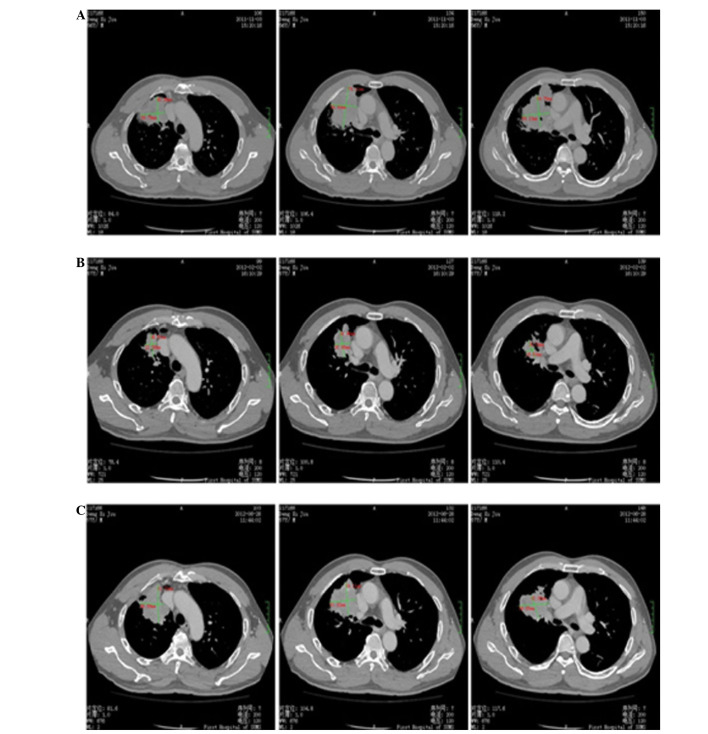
Computed tomography scans of the present non-small cell lung cancer patient. (A) Prior to treatment with gefitinib, (B) stable disease and (C) disease progression following long-term treatment with gefitinib.
